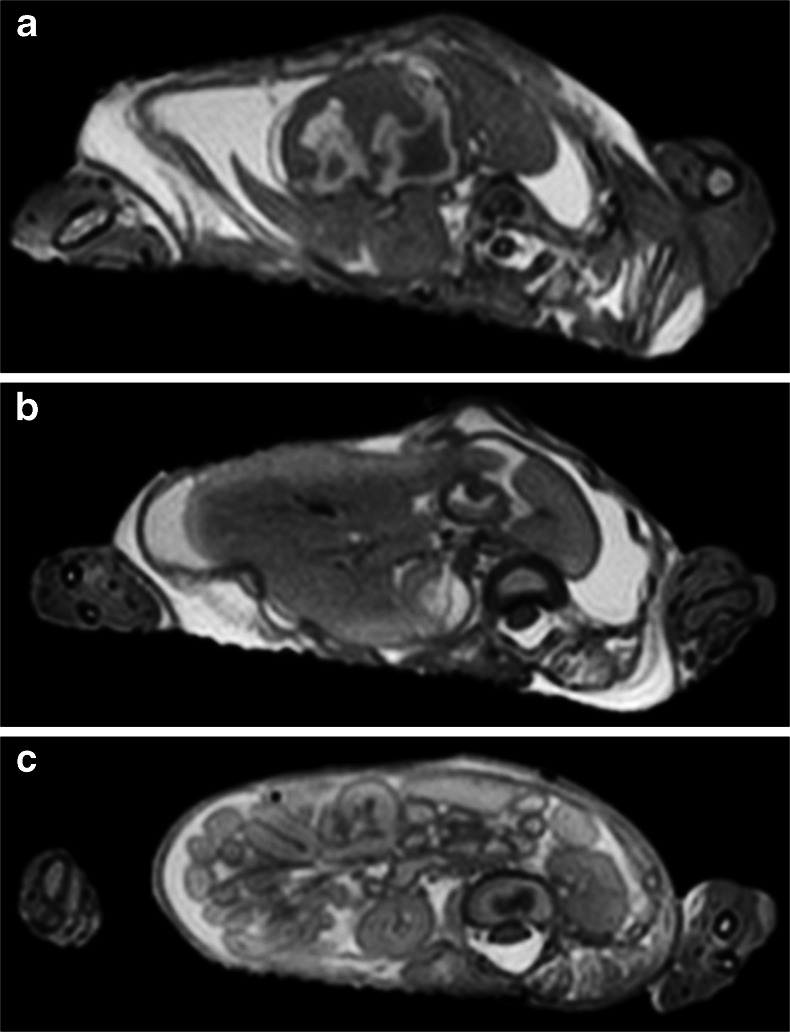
Body organ shifting. Axial T2-weighted postmortem magnetic resonance imaging of a 27-week gestation unexplained intrauterine death, demonstrating organ shifting of the body to one side, affecting the thoracic (a) and abdominal (b and c) compartments. This leads to overinterpretation and misdiagnosis: Both malrotation and asplenia were initially reported in this case prior to 3-D reformatting, as the spleen was difficult to visualise separate from the liver (b) and small bowel loops were predominantly right-sided (c). No anatomical abnormality was identified at full autopsy in this example. Subcutaneous oedema, but no skin slippage, is apparent
